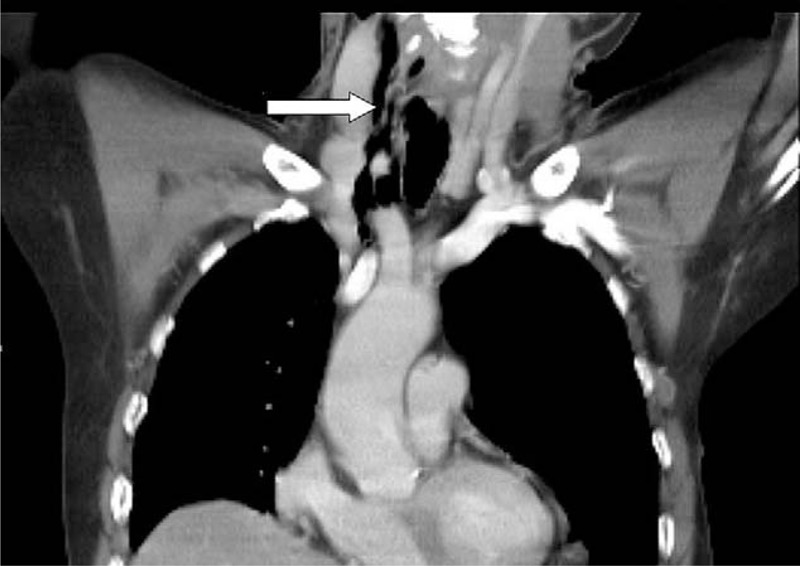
Coronal view of thoracic-CT revealed pneumomediastinum extending upward into the right lower neck region (arrow). CT = computerized tomography.
